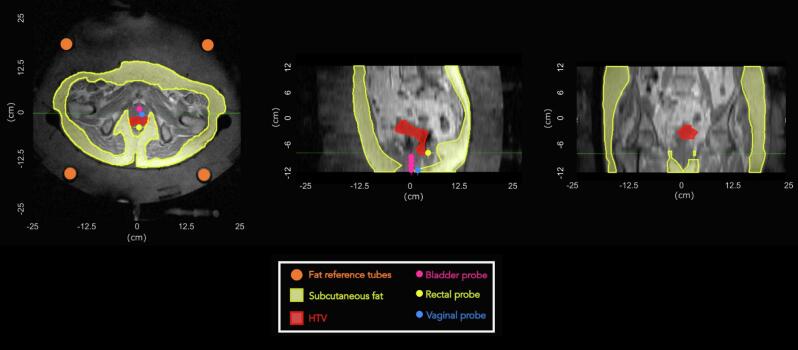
Axial (left) and sagittal (middle) and coronal (right) views of the T1-weighted MR image. Fat reference tubes and subcutaneous fat are delineated in orange and yellow, respectively. The delineation of the HTV is shown in red, while the locations of the thermal probes are shown in magenta, yellow and blue, for bladder, rectal and vaginal probes, respectively. (HTV: hyperthermia target volume). (For interpretation of the references to colour in this figure legend, the reader is referred to the web version of this article.)
